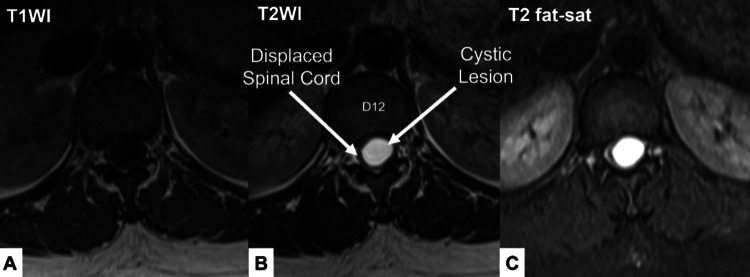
MRI of the patient - image 2 Axial images through the body of the T12 vertebra with T1-weighted (A), T2-weighted (B), and T2 fat-saturated (C) sequences showing a cystic lesion in the thoracic spine. The lesion is hypointense on T1 and hyperintense on T2 and T2 fat-saturated images MRI: magnetic resonance imaging
